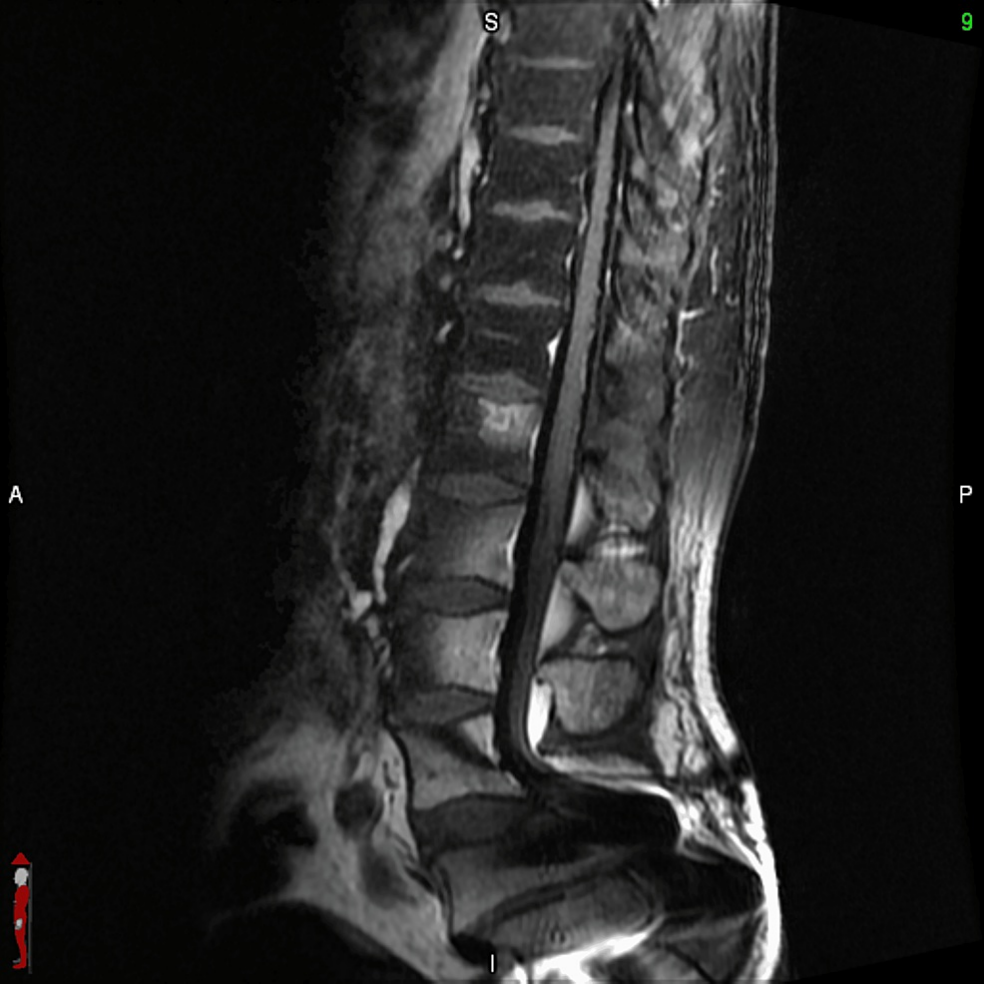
Sagittal spine MRI T1 FLAIR-FS from T8 to S1 showing no abnormal enhancement or signs of leptomeningeal disease (image degradation caudal to the level of L4-5 onwards due to metallic rods and screws) FLAIR-FS: fluid-attenuated inversion recovery with fat suppression
